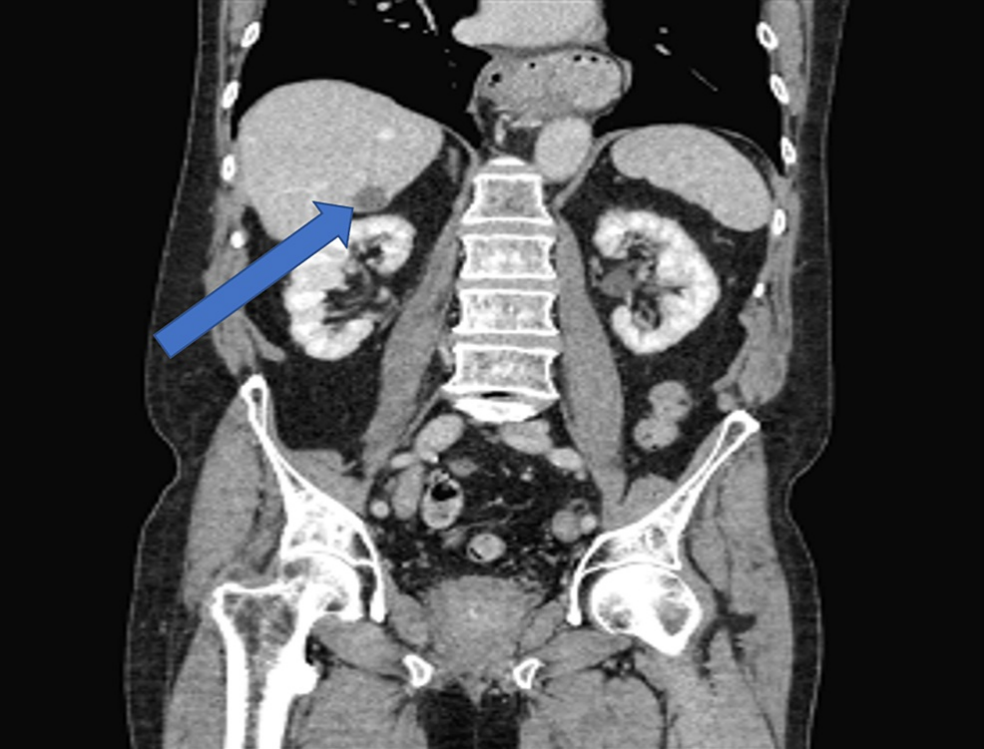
CT abdomen and pelvis triple-coronal view phase demonstrating a 2.6-centimeter lesion in liver segment 6
